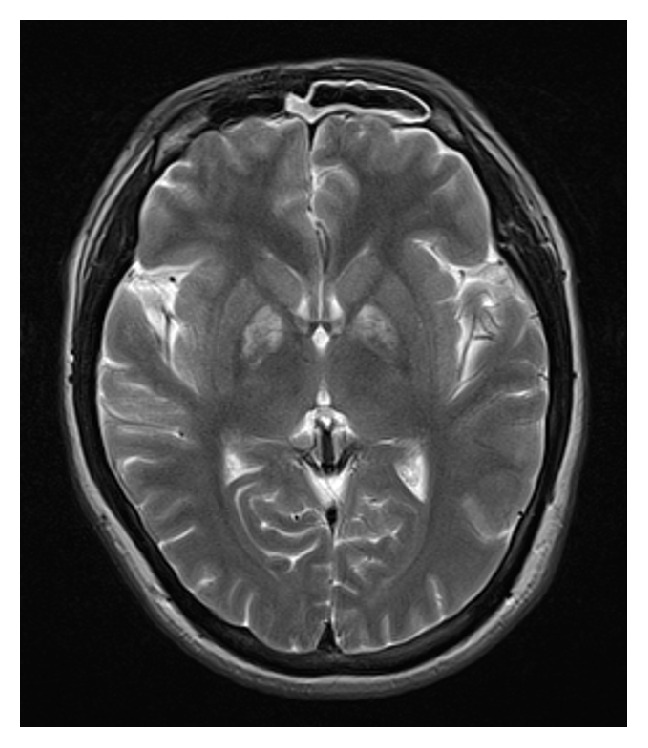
Axial T2-weighted MRI image in a patient with severe methemoglobinemia shows increased signal intensity bilaterally in the globus pallidus.
